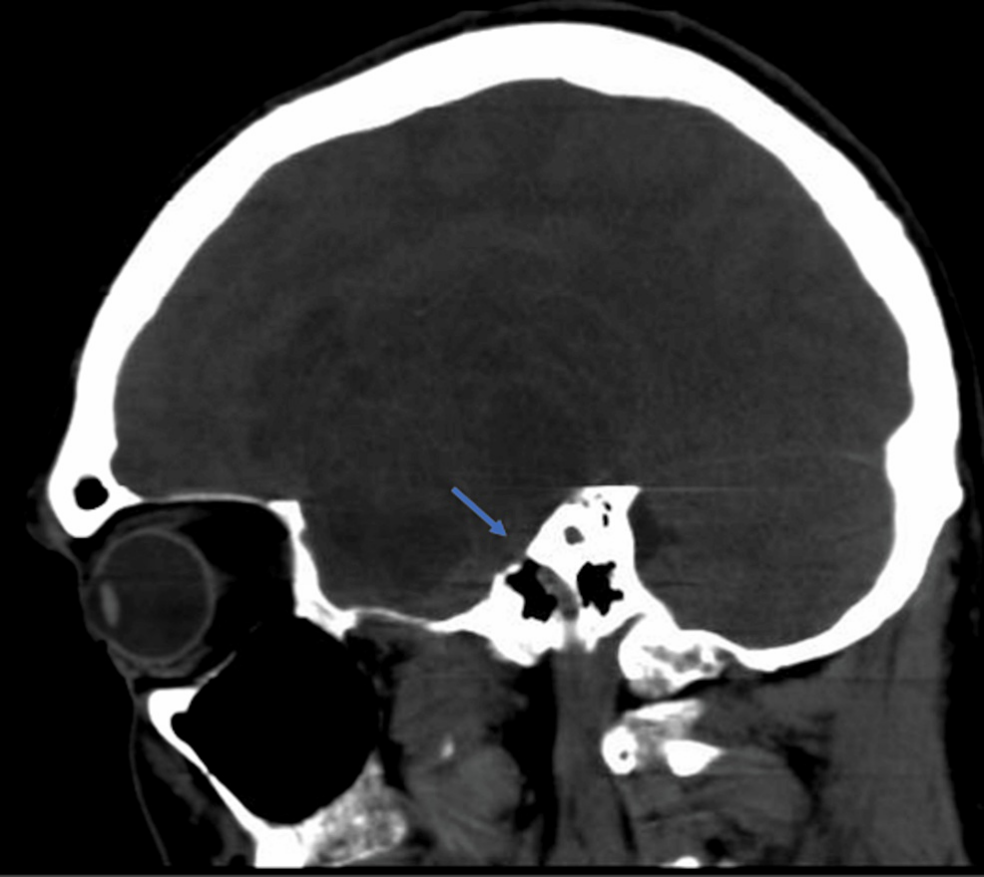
Preoperative CT head: sagittal view without contrast The view shows the mass emanating from the superior aspect of the petrous temporal bone.
